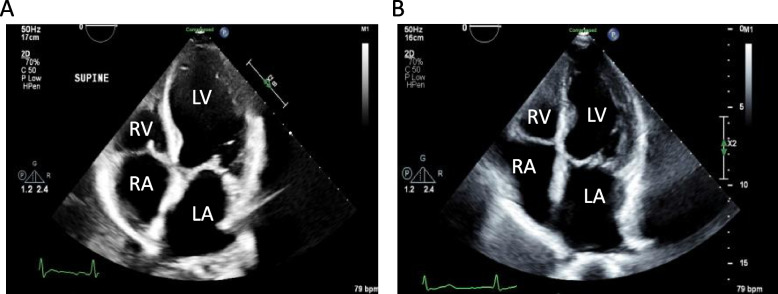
Transthoracic echocardiogram of patient in four chamber view during admission (A) and 2 months prior to admission (B) with representative chambers labeled
